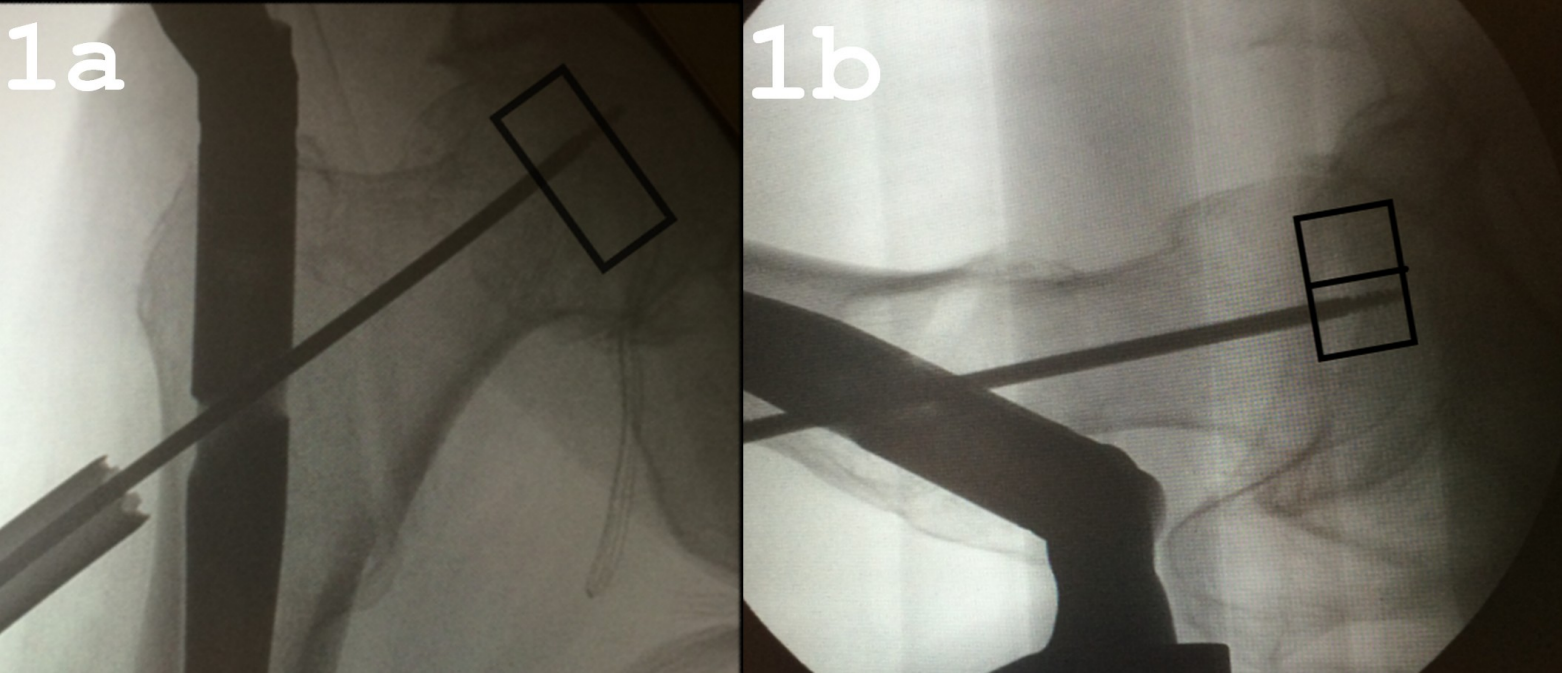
The intraoperative radiographs of intertrochanteric fracture fixation. The shapes drawn in the radiographs show the relative size, shape and direction of the cut bone samples as well as the direction of the hole drilled with the guide wire. (a) anteroposterior view. (b) axial view.
